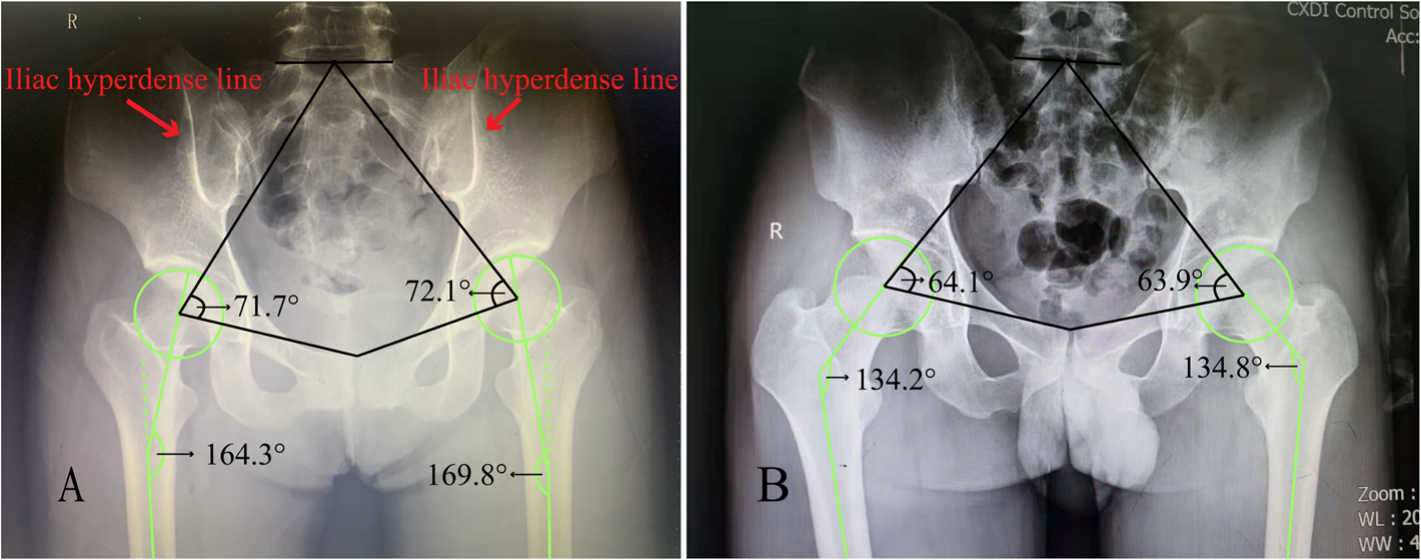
A: AP pelvic radiograph of a patient with GMC; red arrow, iliac hyperdense line. B: AP pelvic radiograph of a patient without GMC
